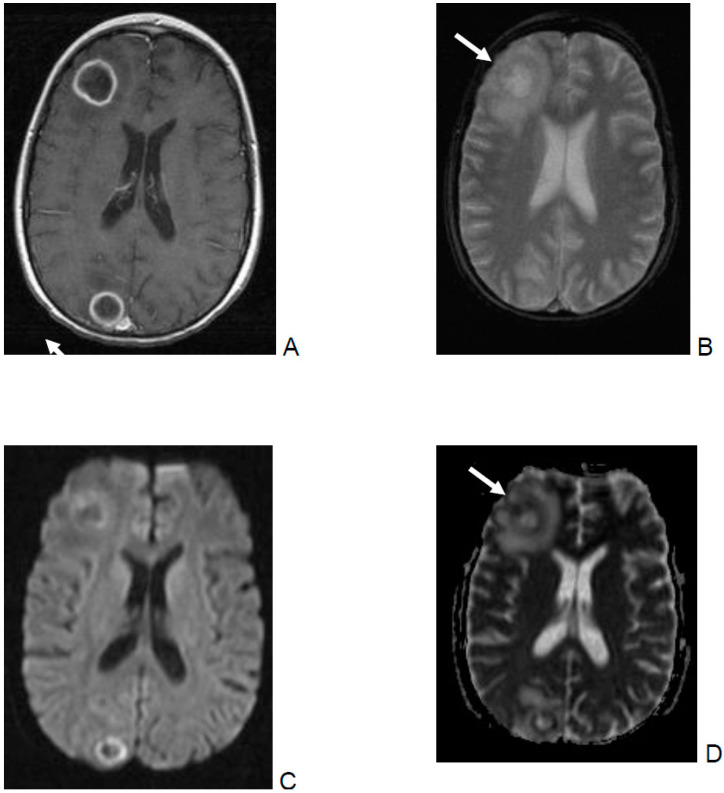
Multiple abscesses in the right hemisphere of a 15-year-old boy with non-Hodgkin lymphoma. Culture grew *Aspergillus fumigatus*. The patient is alive with no neurological sequelae. Two lesions are seen with a slightly hypointense wall on axial T2* image (B), which show well-defined ring enhancement on the post-contrast T1-W image (A). Diffusion, b = 1000 s/mm^2^ (C) shows ring-like hyperintensity with low ADC (0.54 × 10−3 mm^2^/s) (D). Note that fungal abscesses may have a variable imaging appearance but are often multiple and near the grey–white matter junction. The pattern of diffusion restriction of the wall without central restriction correlates more with a fungal abscess than a pyogenic abscess. On DWI/ADC (C and D) the walls as well as the small nodular soft tissue (straight arrows) display diffusion restriction, while the contents do not show restriction. There is perilesional edema.
